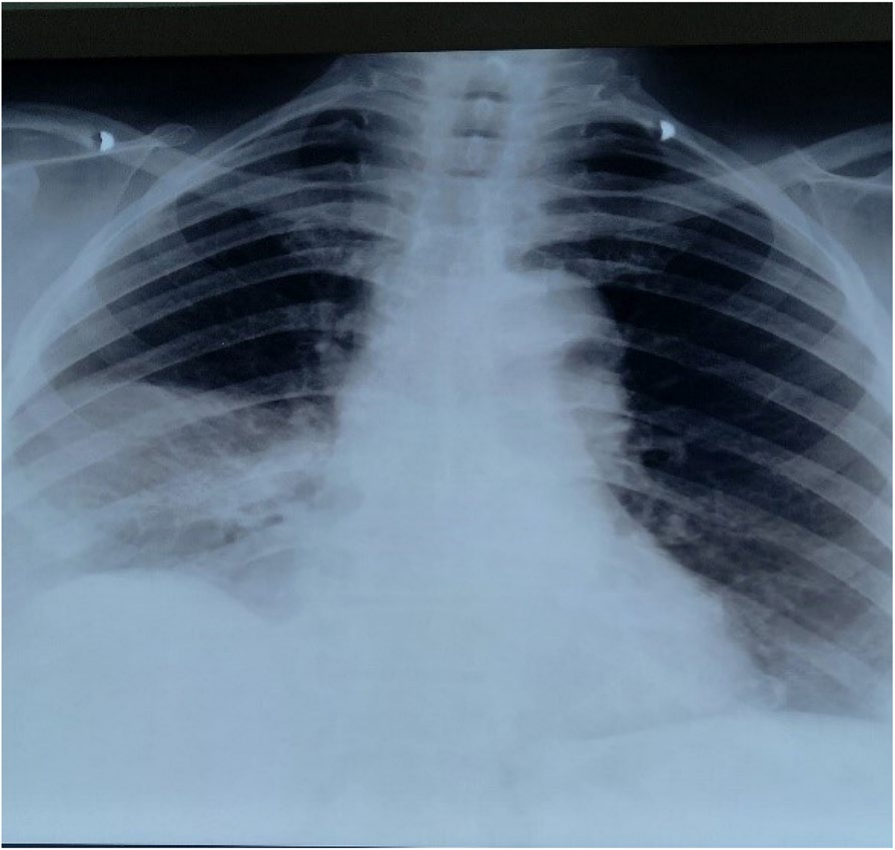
Chest X-ray showing homogeneous opacity on the right middle and lower lung zones and elevated right hemi-diaphragm
